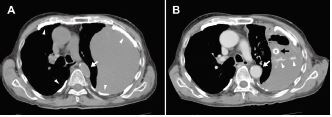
Computed tomography (CT) at the level of the tracheal bifurcation (A) before and (B) after chest drainage. (A) Plain CT at the level of the tracheal bifurcation shows mediastinal shift toward the right, compression of the descending thoracic aorta (arrow), massive left hemothorax, and multiple pleural plaques (arrowheads). (B) Contrast-enhanced CT after decompression of the left thorax shows improvement in the aortic compression (white arrow), a contrast blush indicating intrathoracic hemorrhage (arrowheads), and a drainage tube (black arrow) within the left-sided pleural effusion.
